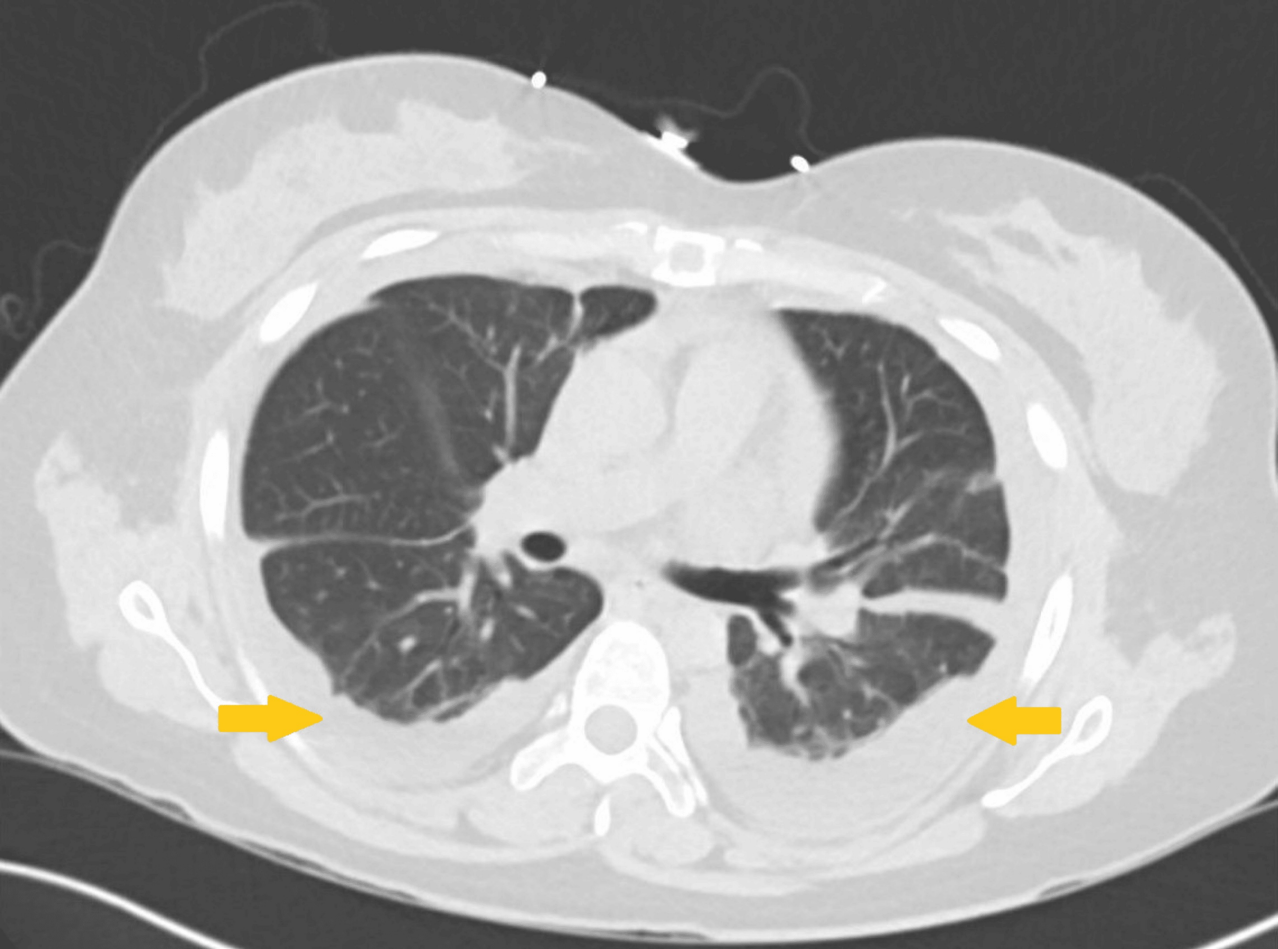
A CT scan of the chest without contrast from initial hospitalization showed bilateral pleural effusions.
